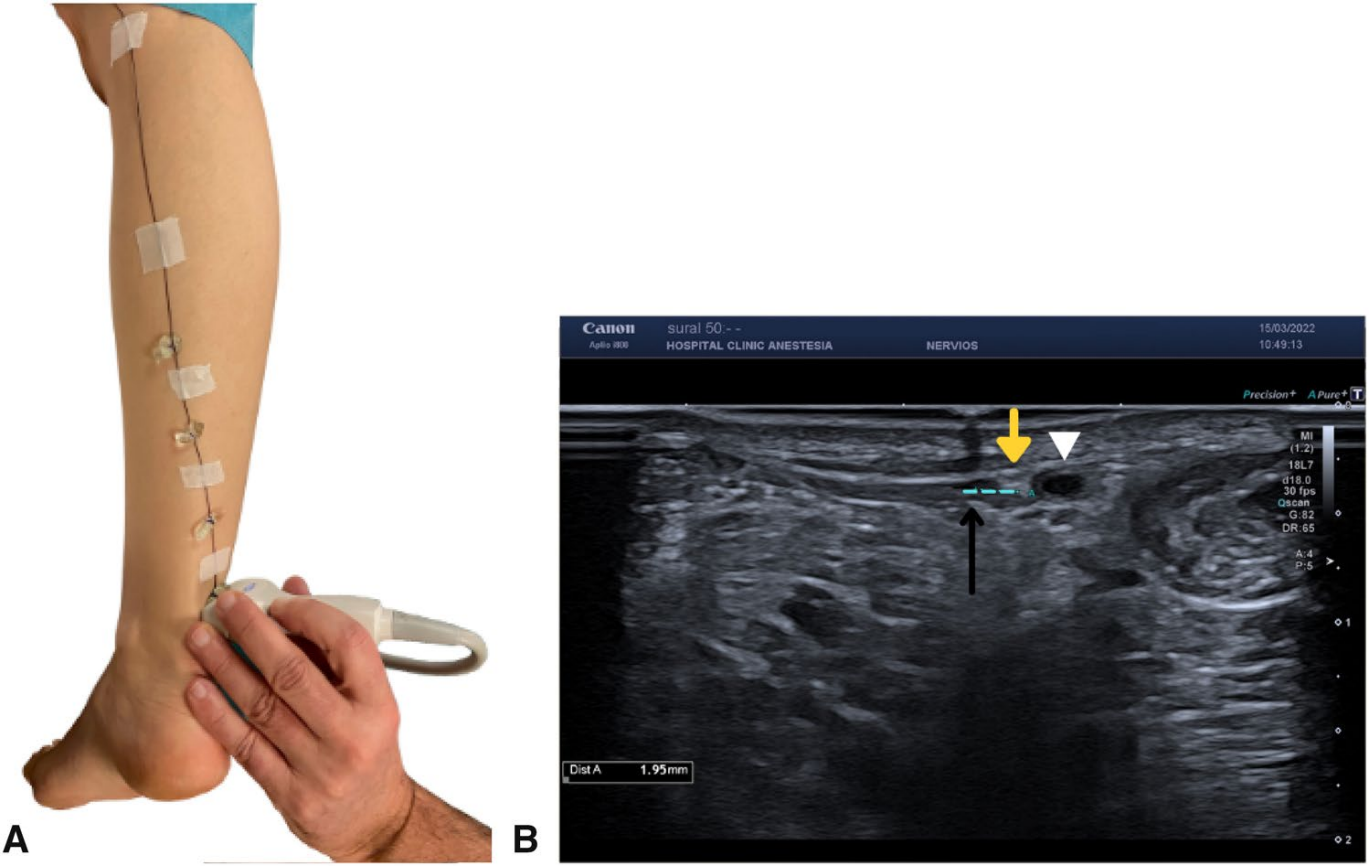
Sural nerve ultrasound evaluation and its relationship with surface reference line on a left leg. A Clinical position of the sonography for the evaluation of the sural nerve in relation to Point 1. B Sonographic image shows the distance (dotted light blue line) between the sural nerve (yellow arrowhead) and the acoustic shadow of the line (black arrow). White triangle: saphenous vein
